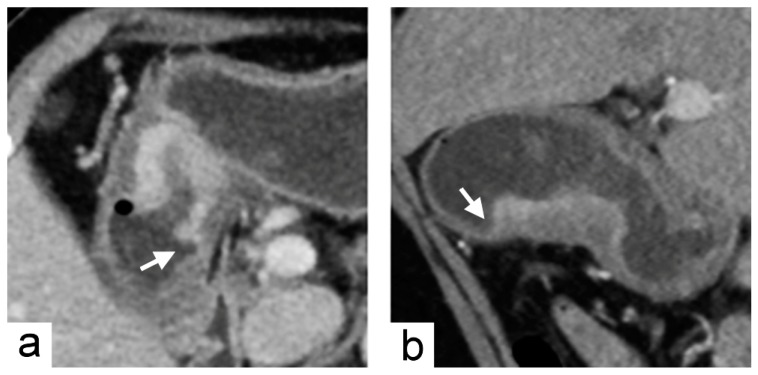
Chromosomal instability subtype gastric cancer in a 79-year-old female who underwent preoperative contrast-enhanced computed tomography. (a) portal venous phase axial and (b) arterial phase sagittal images showed focal wall thickening of the greater curvature side of the stomach with the largest diameter of 3.1 cm. In the imaging traits evaluation, the tumor morphology was defined to be acute tumor transition angle (arrows in (a,b)), well-defined margin and polypoid shape.
